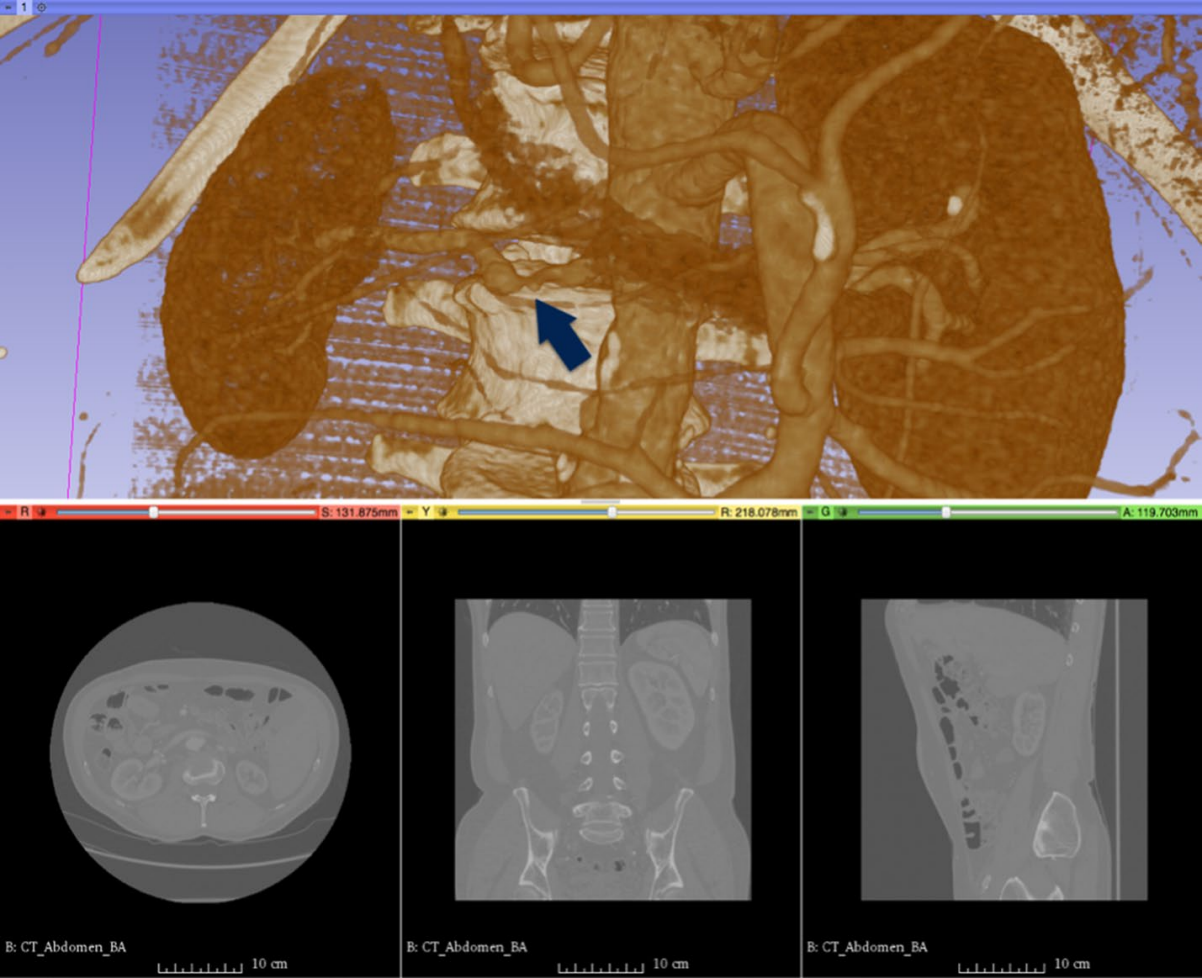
CT data set for a patient with severe stenosis in the left renal artery (blue arrow)—axial, sagittal and coronal slices (bottom row) and volume reconstruction (top panel). Image visualization performed using Slicer 3D software [30]
